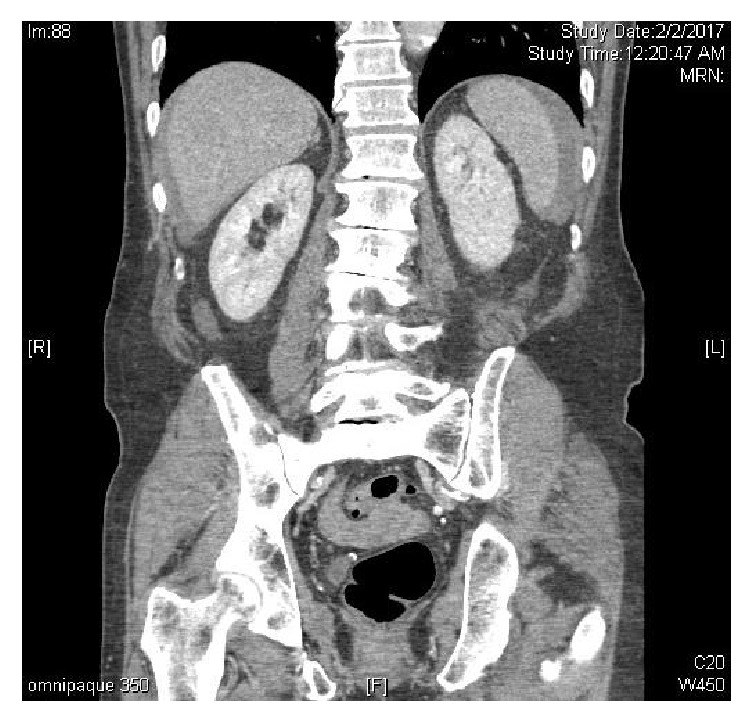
Coronal CT scan demonstrating hemoperitoneum from iatrogenic spleen injury.
